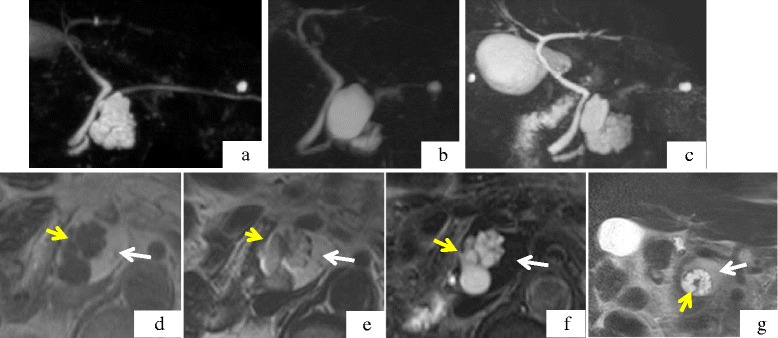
a, b, c. Chronological changes seen on MRCP. a: At the first examination. The tumor was observed as multiple cystic lesions. b: At 21 months after first examination. c: At 28 months after first examination. Figure 2-d, e, f, g. MRI appearance at 28 months after first examination. d: T1WI. A multi-cystic lesion with low intensity was surrounded by a mass with iso-intensity. e: T2WI. A multi-cystic lesion displays low- to iso-intensity. The surrounding tissue shows iso- to high intensity. f: T2-FAT-SAT. A multi-cystic high intensity lesion is shown. The surrounding tissue shows complete fat suppression. g: T2WI (coronal image). A ringed multi-cystic lesion with high intensity is surrounded by a smooth superficial mass. A small nodule is seen inside of the cyst ring
